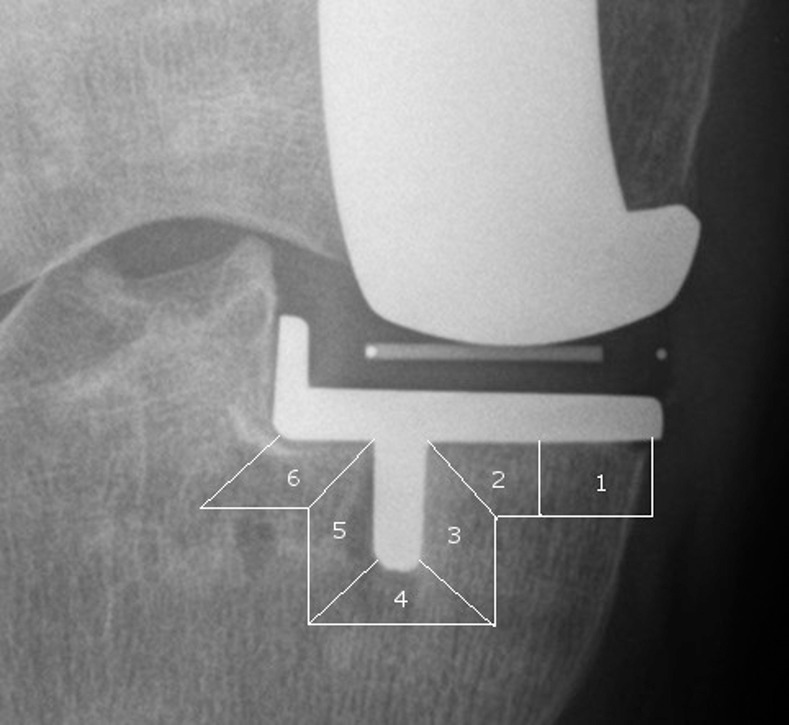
Zones of radiolucent lines on an a.p. radiograph.
